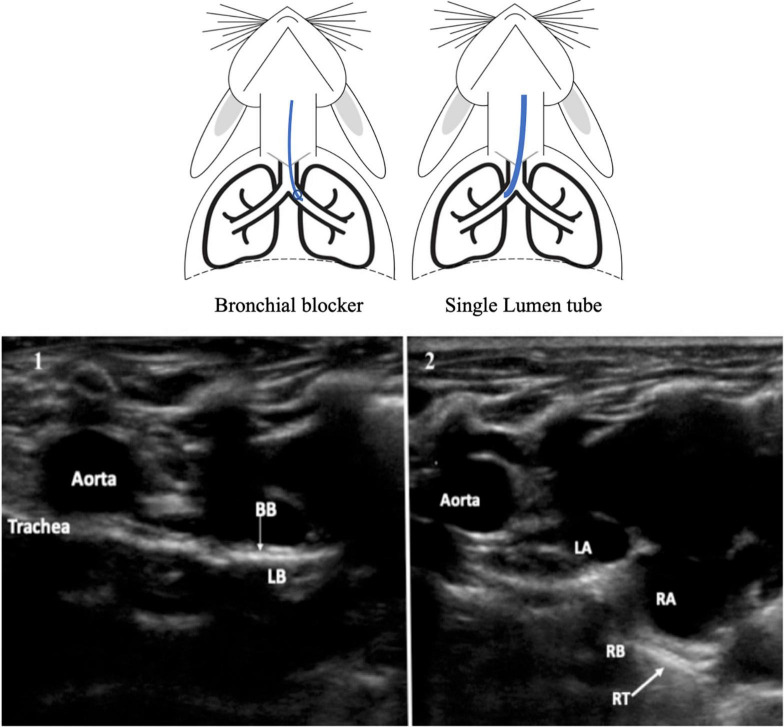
Oblique, parasternal, and midclavicular plane 1. Bronchial blocker in the left bronchus. BB, Bronchial blocker, LB, Left bronchus. 2. Reinforced tube in the right bronchus. RT, Reinforced tube, RB, Right Bronchus, LA, left atrium, RA, right atrium.
